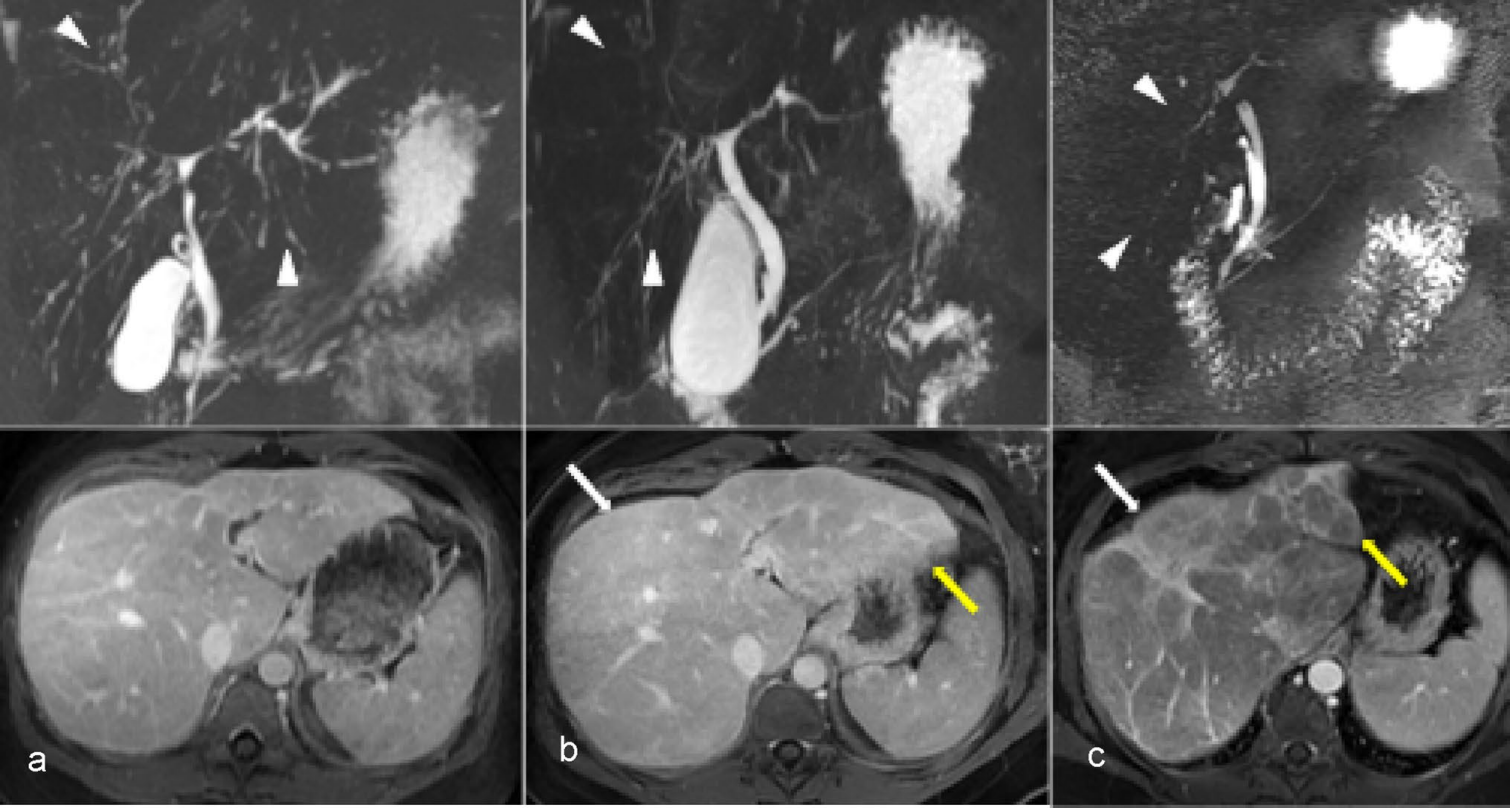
A 29-year-old female with Ulcerative colitis since the age of 14 years old presents with cholestasis. Magnetic resonance cholangiopancreatography (MRCP) maximum intensity projection and axial T1-weighted post-contrast images at the time of diagnosis (image a); at 4 years (image b) and at 12 years (image c) are shown. The MRCP demonstrates classic stricture-dilatation of the intrahepatic bile ducts (arrowhead, a). With disease progression, there is progressive obliteration of the bile ducts (arrowheads, b–c). The liver also shows progressive decrease in volume (yellow arrows, b–c), worsening of fibrosis (white arrow, b–c) and development of cirrhosis
